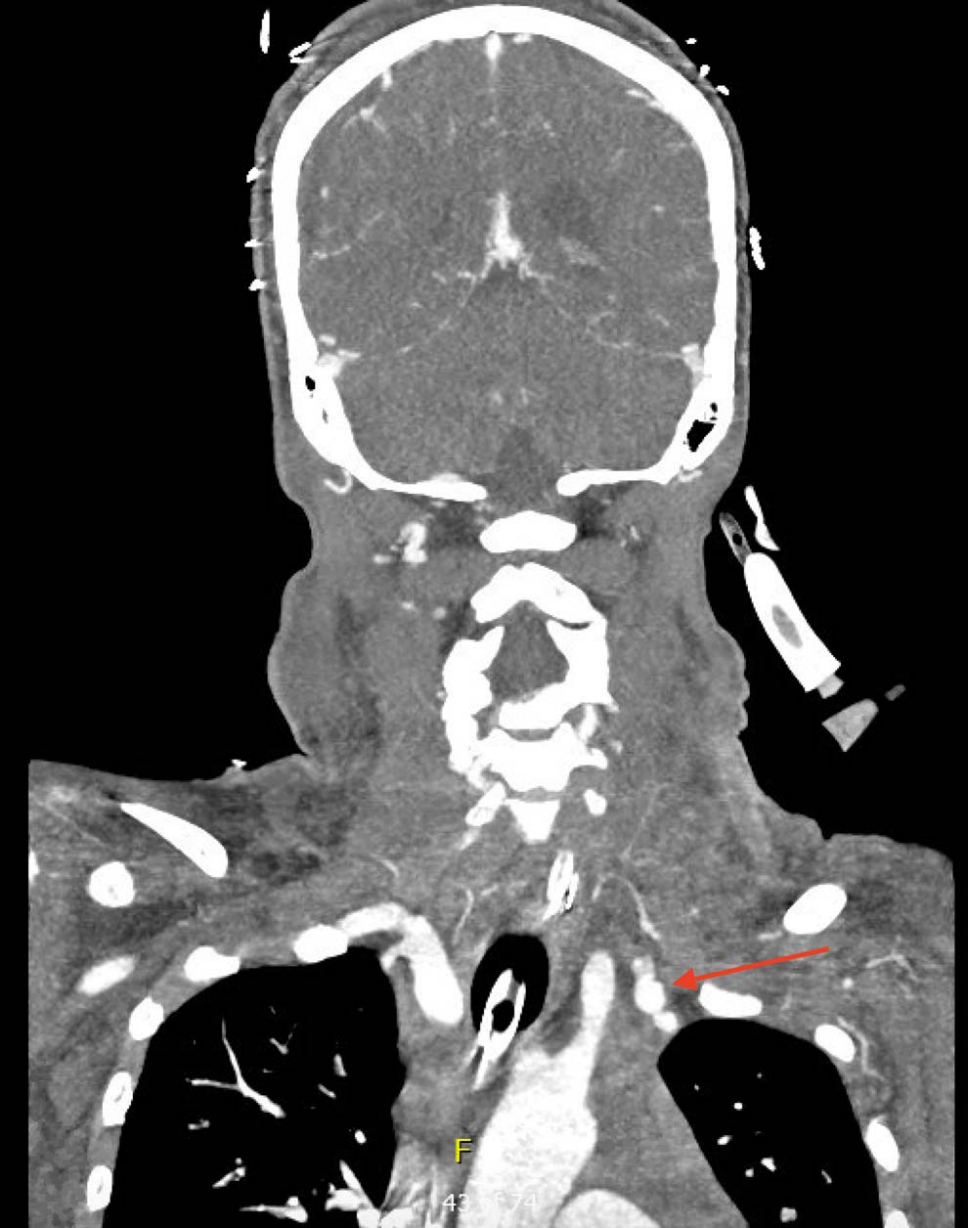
Computed tomography with contrast of the head and neck on day 2 (coronal view) found a dilated left internal mammary artery with possible bleeding (arrow).
